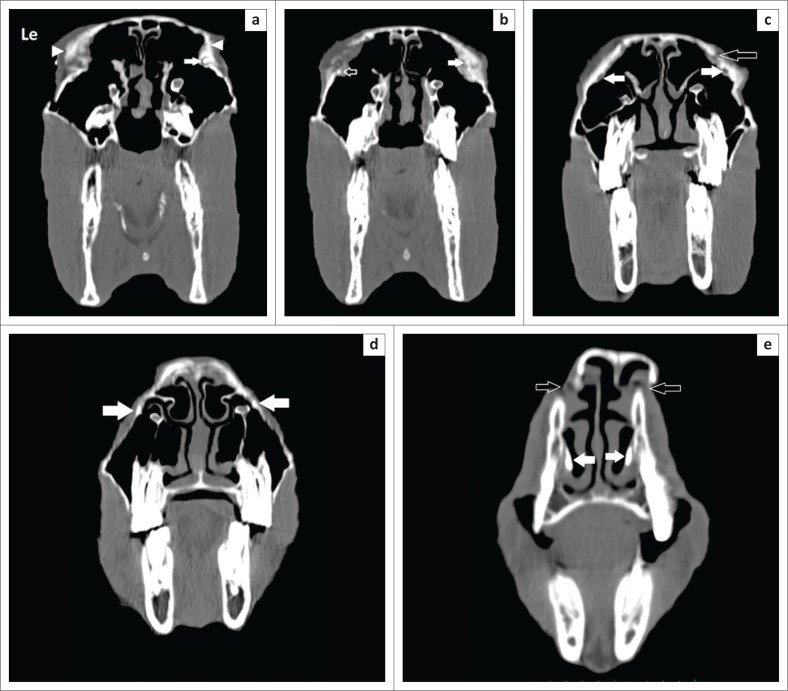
A sequence of slightly oblique transverse computed tomography images are presented from caudal (level of the medial canthus of the eyes) (a) to rostral (e). In (a), the contrast medium is present within the right ventral nasolacrimal puncta (white arrow) but is not visualised on the left. Note the marked bilateral lacrimal bone periosteal proliferation present (white arrowheads). In (b), the contrast material is not clearly visualised on the right (white arrow), in the region of the most severe bony proliferation, but is present on the left as a focal region of hyperattenuation (open arrow). In (c), at the level of the caudal maxillary molars, the contrast material is not visible in either nasolacrimal duct (white arrows), and a focal cortical defect in the lacrimal bone is visualised. In (d), a small amount of contrast attenuation can be visualised within both nasolacrimal ducts (white arrows) with the contrast present in the nasal passages (white arrows) in (e), immediately caudal to the nasal puncta. Note the discontinuity and displacement of the dorsal-most aspect of the nasal bones (open arrows), which is because of patient motion and thus is artefactual. The left (Le) of the patient is on the left of the image, and the dorsal is to the top. Images were obtained under standing sedation, in a bone window (WL: 450 HU and WL: 1500 HU).
